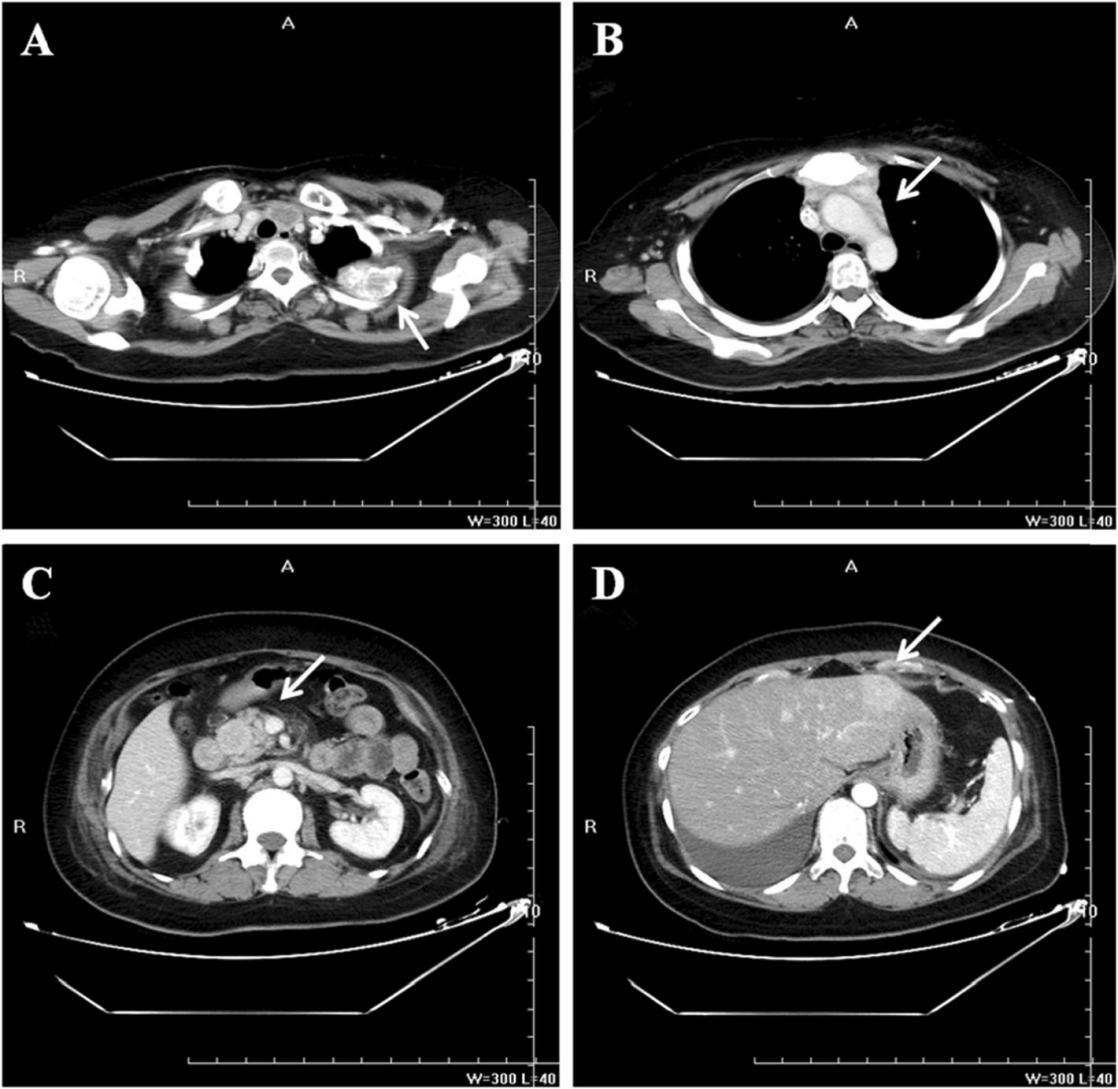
Contrast enhanced computed tomography scan of the upper abdomen show a heterogeneous mass (white arrow) and a high degree of enhancement in the ribs (a), anterior mediastinum (b), pancreas (c), and liver (d)
